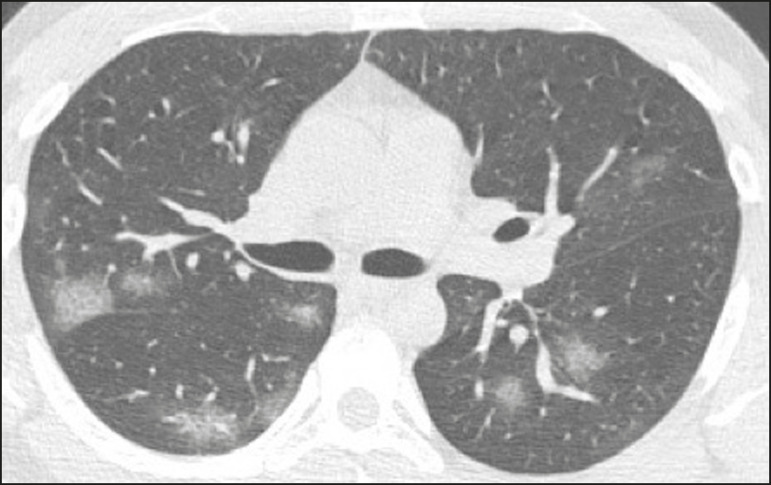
Chest CT scan of a 34-year-old female patient with a 4-day history of chest pain and cough who tested positive for SARS-CoV-2 on RT-PCR, showing bilateral ground-glass opacities with a predominantly peripheral distribution.
